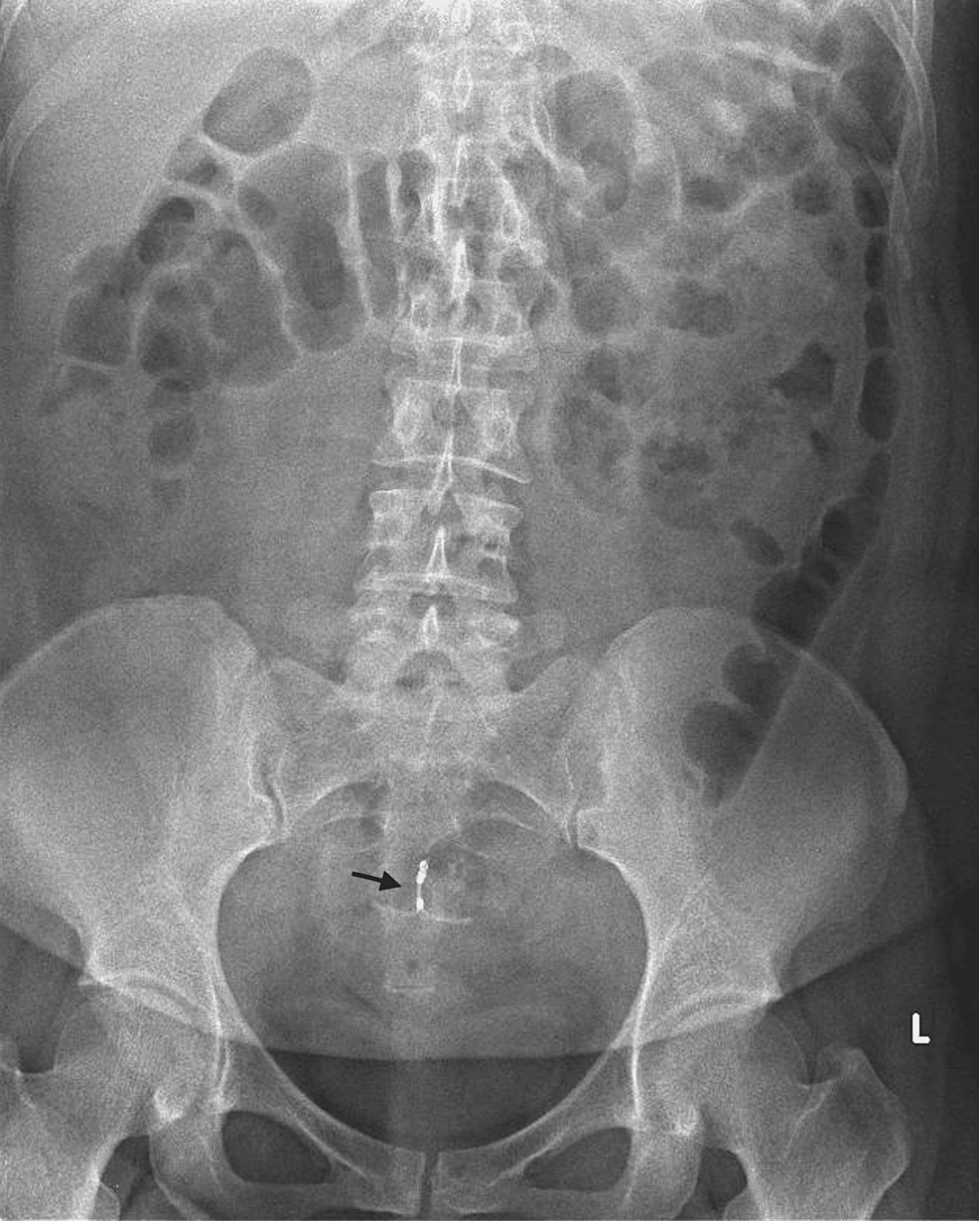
An X-ray of the KUB shows an intrauterine device (arrow) noted within the midline of the pelvis. No renal stones were noted. KUB: kidney, ureter, and bladder
